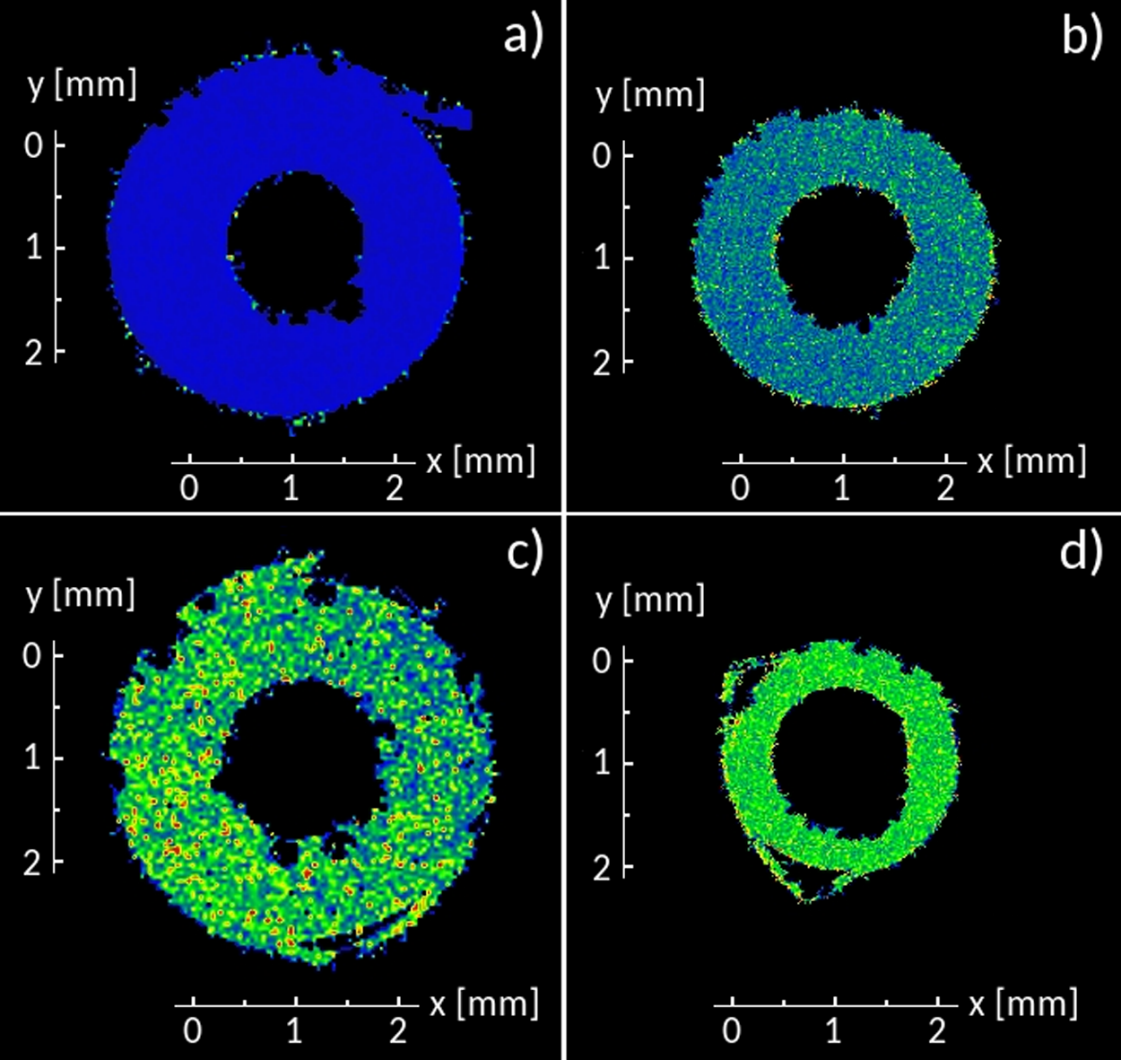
T*_2_-weighted MRI images for the samples:
